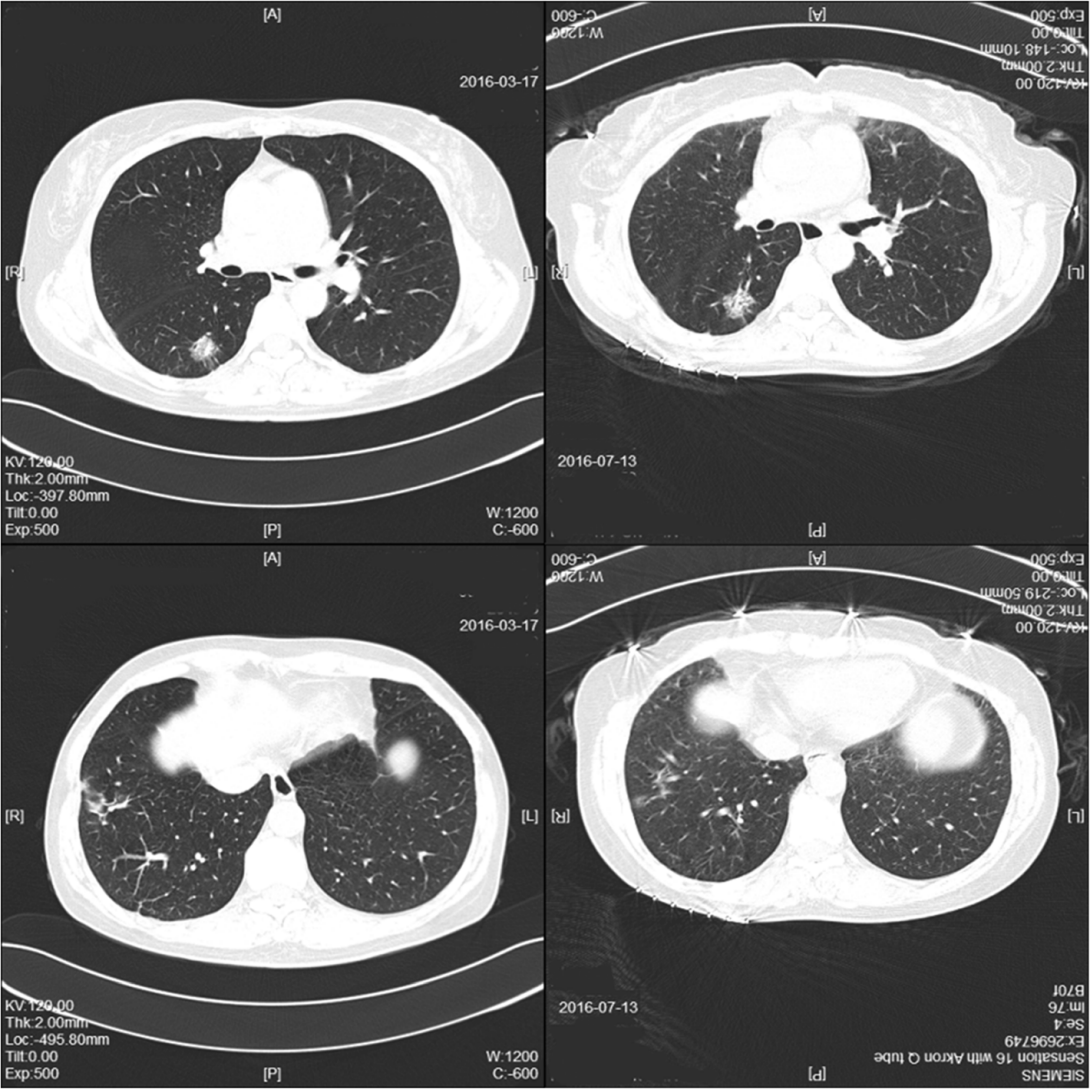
Two months after an operation using cannulated screw internal fixation for the patient’s femoral neck fracture, computed tomography showed that the size of the lesion in her right posterior segment had increased, though the lesion in her right lateral basal segment had decreased
